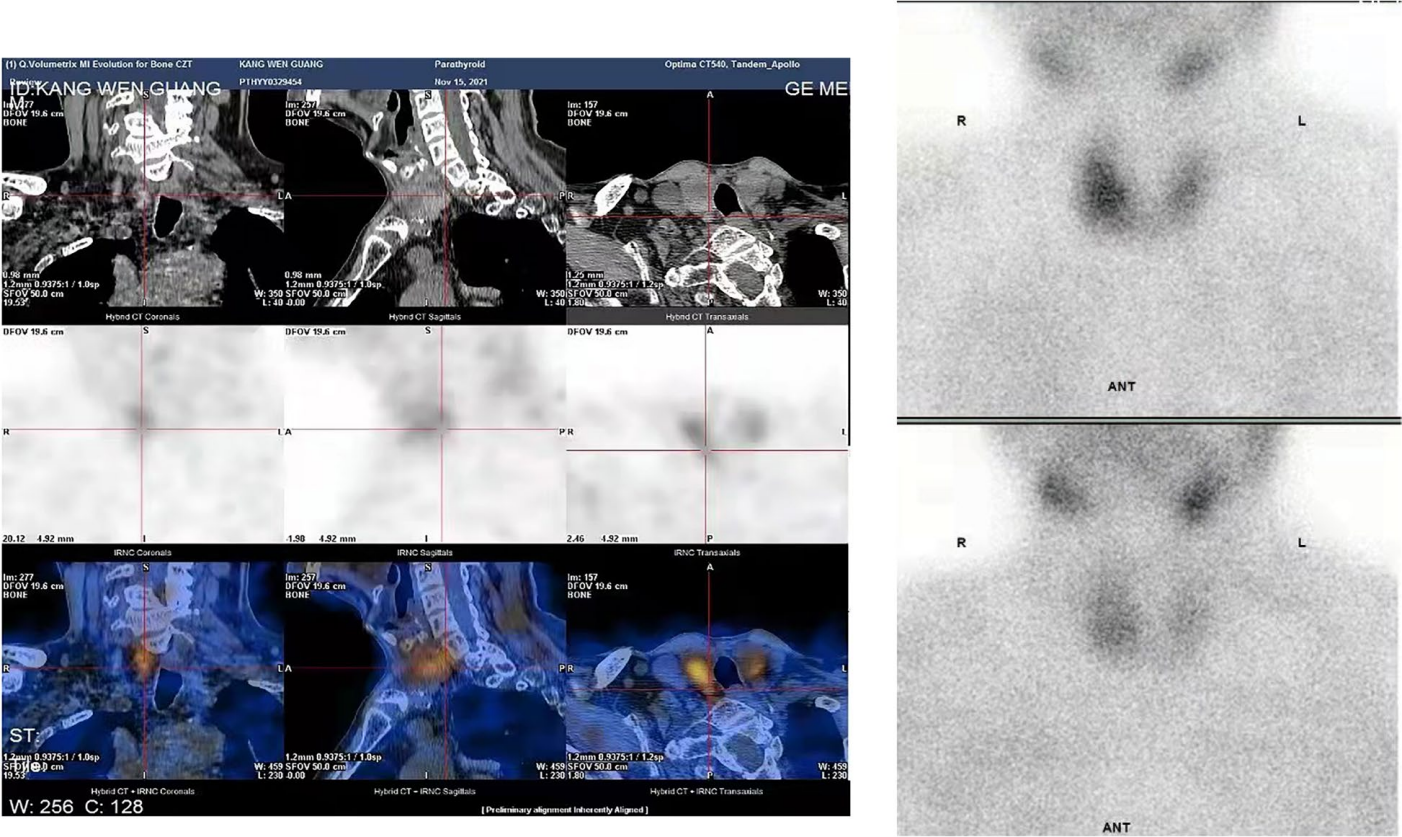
30 min after intravenous injection with 99mTc-MIBI showing an enlarged right lobe of thyroid and a slight hyperactive zone in the middle and lower part. 2 h later, there was persistent radioactive focal in the middle and lower part of right lobe. A SPECT scan revealed an enlarged thyroid gland in the right lobe, with a focal area (7.2 × 8 mm) in the middle and lower part
